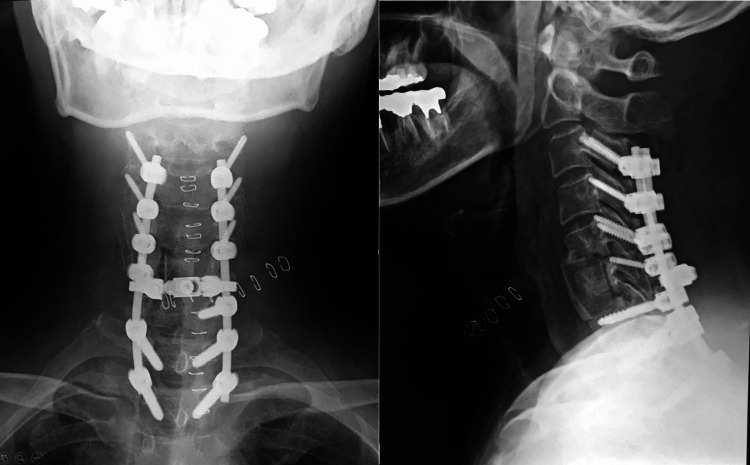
Postoperative X-ray C6 corpectomy with iliac bone graft and posterior spinal fusion from C3-T2 was performed while taking care to avoid hyperlordosis so as to prevent postoperative dysphagia and C5 palsy.
